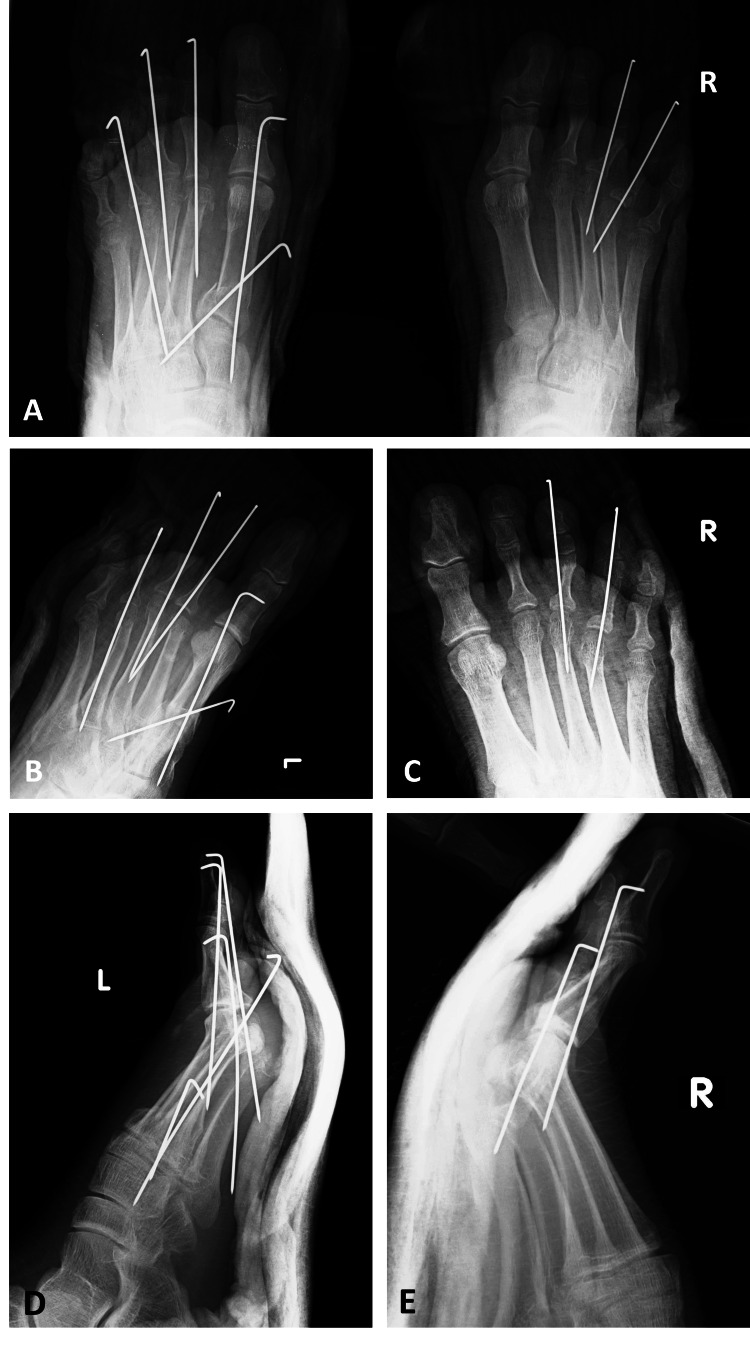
The left and right foot's X-ray views directly after the surgery A: The left and right foot's anteroposterior X-ray view directly after the surgery showed the open and closed reduction with K-wire fixation. B: The left foot's oblique X-ray view directly after the surgery showed the open and closed reduction with K-wire fixation. C: The right foot's oblique X-ray view directly after the surgery showed the closed reduction with K-wire fixation. D: The left foot's lateral X-ray view directly after the surgery showed the open and closed reduction with K-wire fixation. E: The right foot's lateral X-ray view directly after the surgery showed the closed reduction with K-wire fixation.
